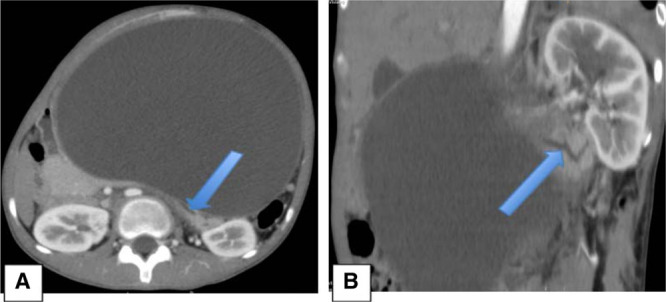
Abdominopelvic CT scan with contrast media in arterial phase, in axial section (A) and oblique coronal reconstruction (B) showing the large cystic mass with dilation of the main pancreatic duct of the caudal portion (arrows).
